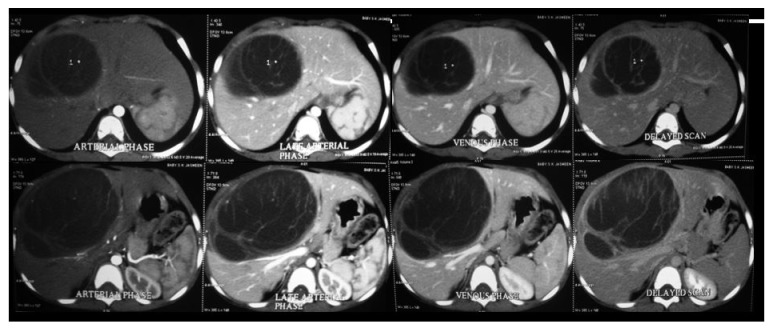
Triple phase CT scan notable for a near water attenuating cyst occupying the central sections of the liver with involvement of the middle hepatic vein. There are few clustered microcalcifications in the cyst.
